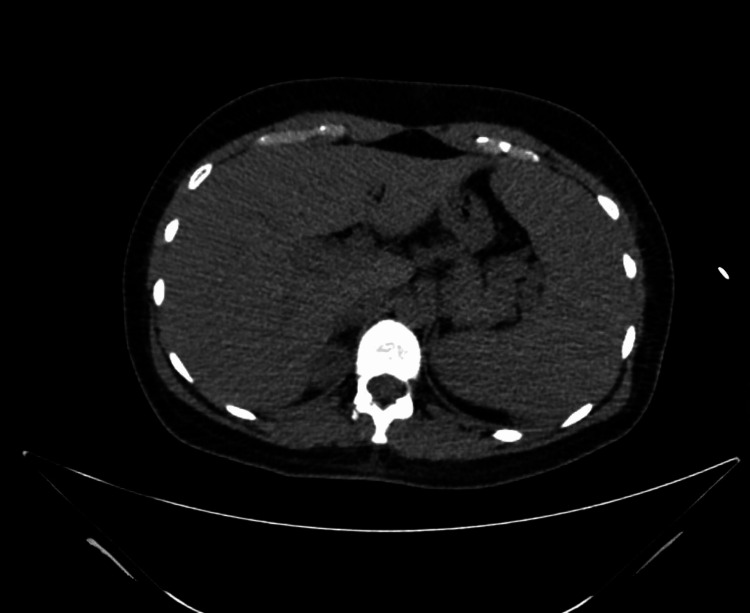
Patient’s non-contrast abdominal CT showing splenomegaly
